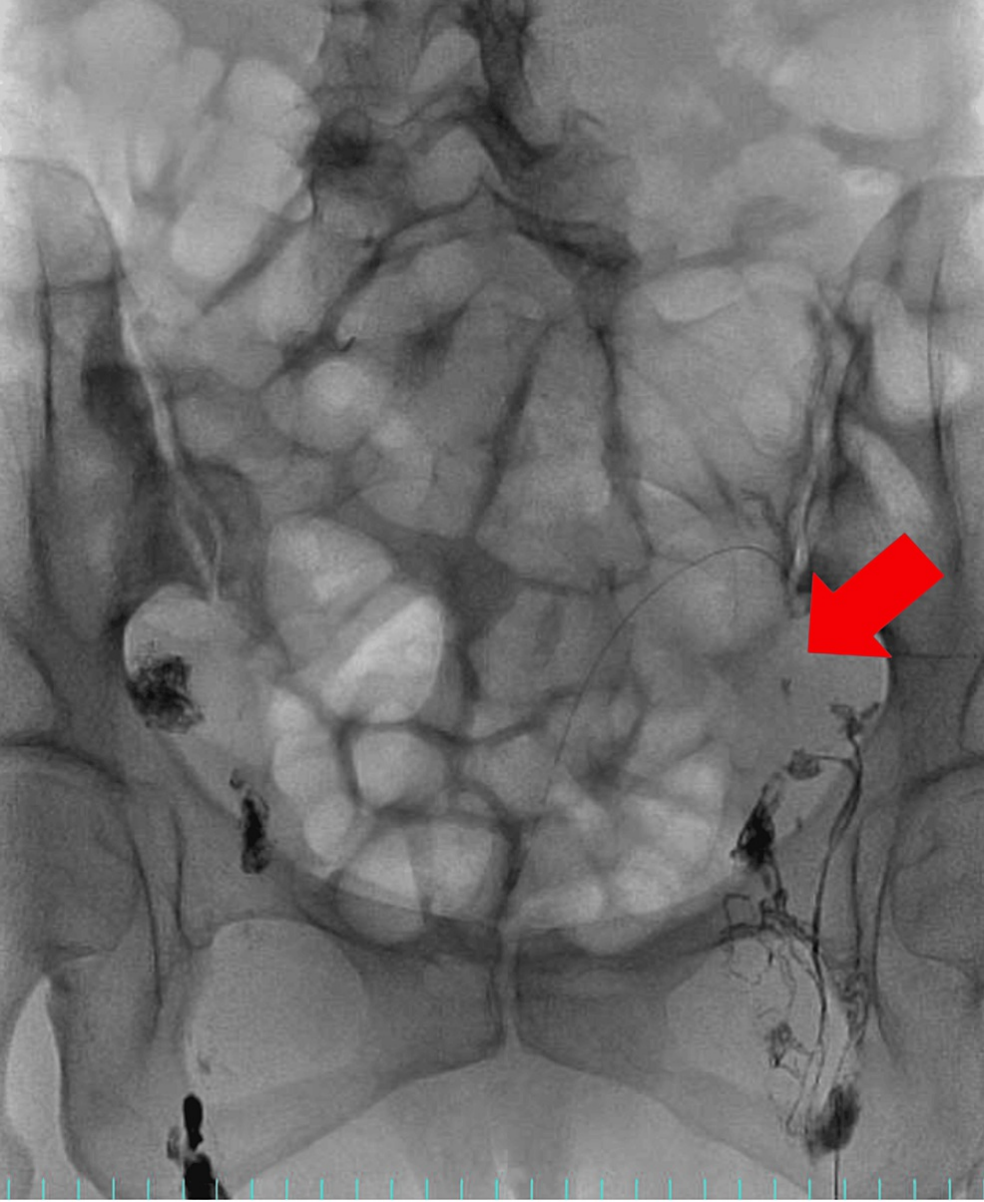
First lymphangiography. It was not possible to confirm that Lipiodol® reached the site of chyle ascites leakage, which had been previously identified by CT. The red arrow shows that the contrast agent could not reach the leak site.
